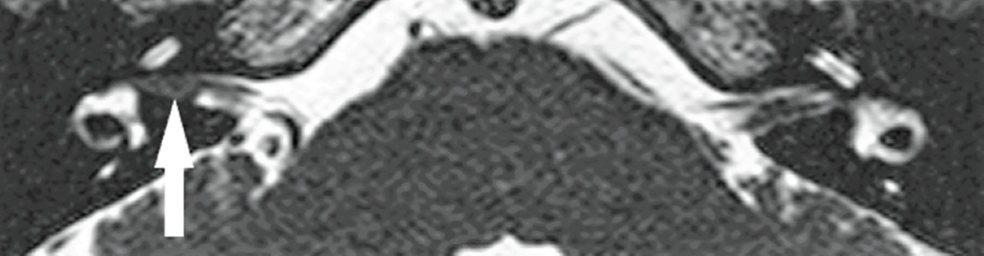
Axial 3D fast imaging employing steady-state acquisition (FIESTA) magnetic resonance image demonstrating a filling defect (arrow) in the right internal auditory canal consistent with a very small vestibular schwannoma.
